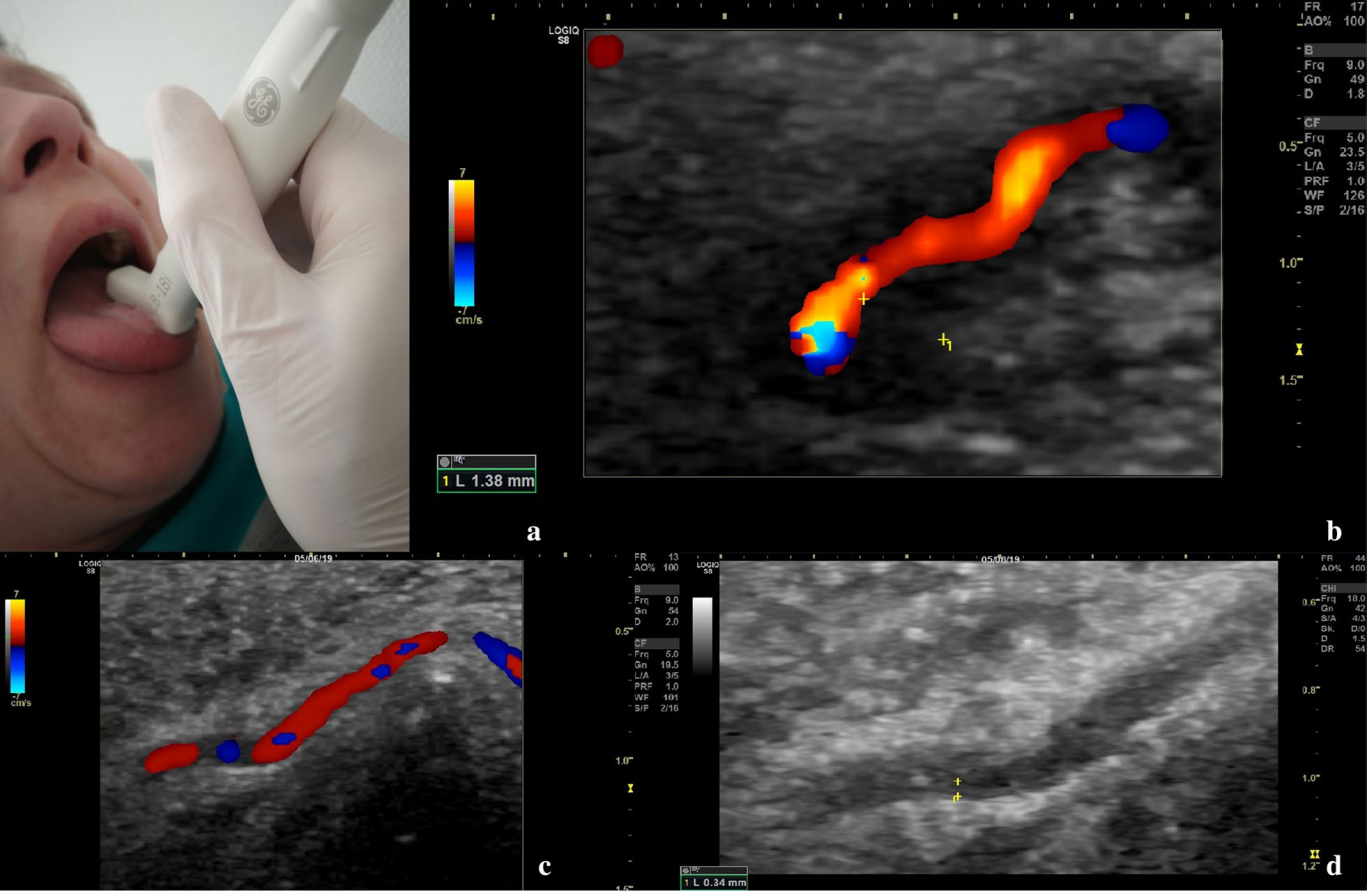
Ultrasound examination of the lingual artery with an 18 MHz hockey stick probe. a Position of the ultrasound probe on the patient´s tongue. b Doppler ultrasound and intima-media thickness (IMT) values of the affected deep lingual artery. c Doppler ultrasound of the deep lingual artery on 6-month follow-up, reduced field of view. d IMT values of the deep lingual artery on 6-month follow-up in B-Mode, reduced field of view
